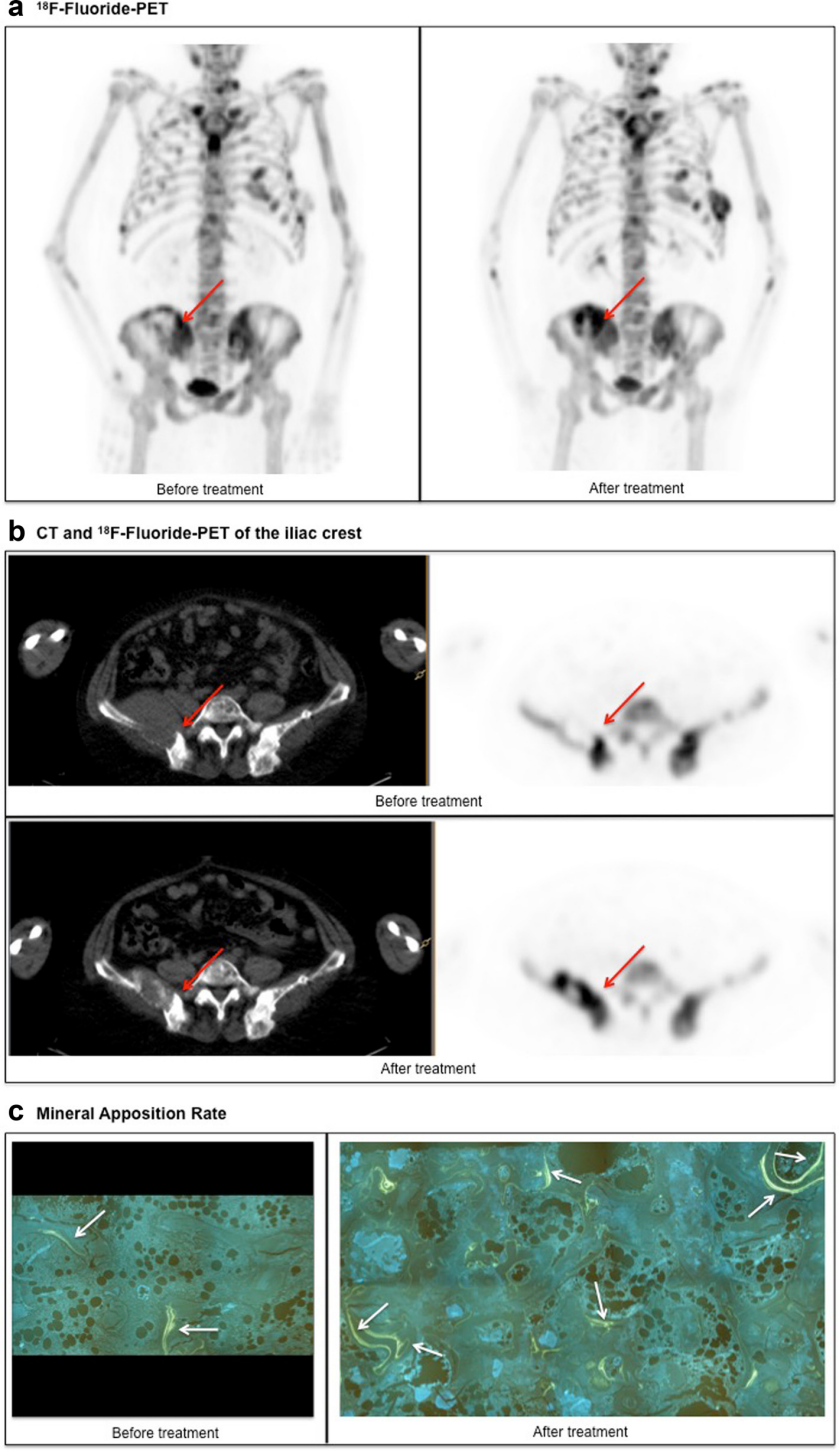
^18^F-fluoride-PET-CT and MAR (patient BBR01). a
